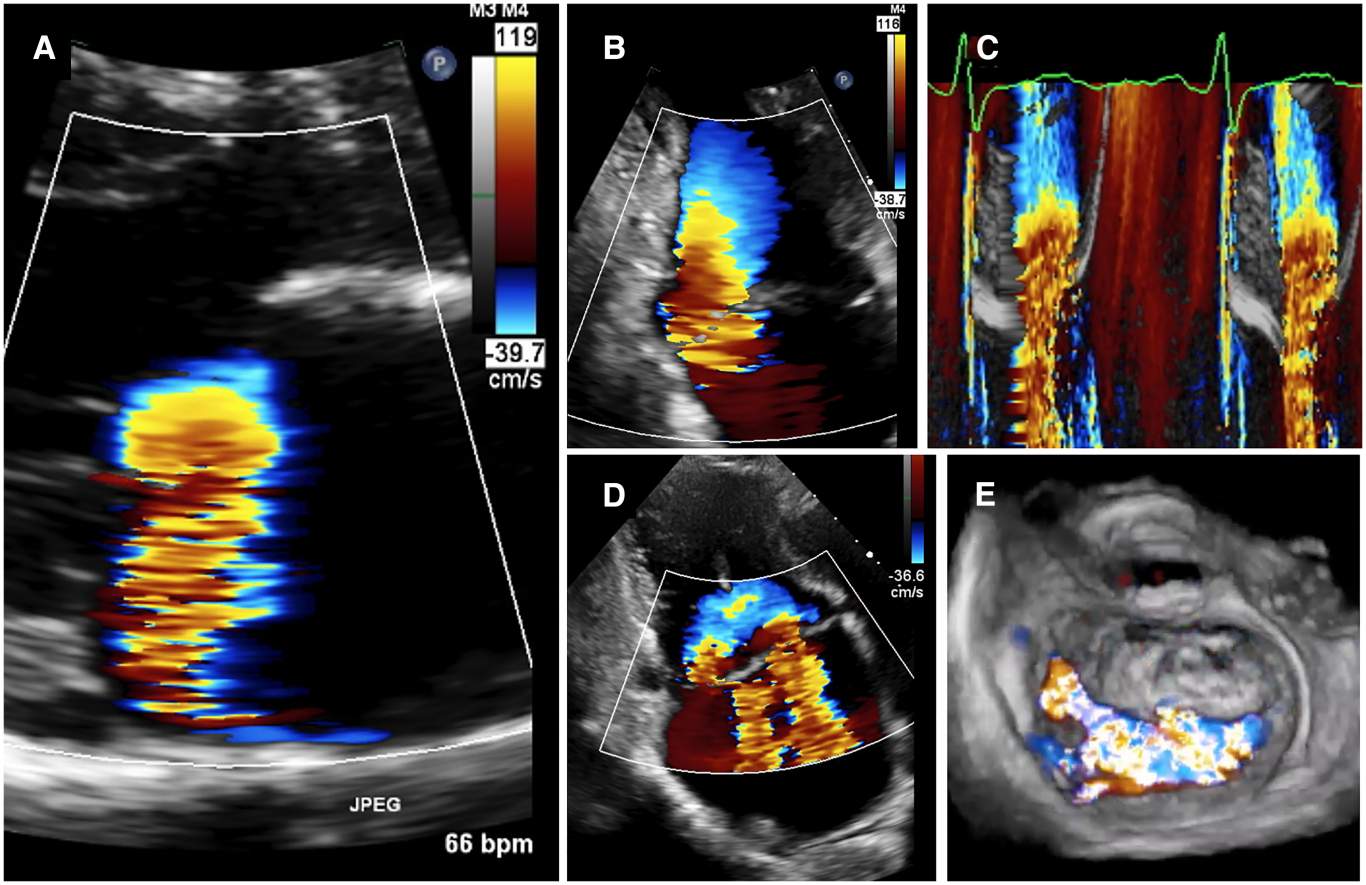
Examples of pitfalls of the PISA method. (A) Patient with anterior MV prolapse. A clear hemispheric-shaped proximal flow convergence is seen on parasternal long-axis view (A). However, this is not the most common situation encountered in clinical practice. (B) Wall-constrained proximal flow convergence with a oblong shape. This is frequently observed in case of posterior MV prolapse or commissural MR. There is a risk of over-estimation of MR EROA and RegVol by the PISA method. (C) Late-systolic MR. As shown in Doppler color coupled to M-mode, the MR is only present in the latter part of systole. Therefore the MR EROA which depends on a single time point measurement (the PISA radius) is not reliable to grade MR. (D) Multiple MR jets. The PISA method is not reliable to grade MR. Indirect quantitative methods should be considered. (E) Bi-leaflet prolapse with extensive MR from one commissure to another. The PISA method is inapplicable. Indirect quantitative methods should be considered. EROA, effective regurgitant orifice area; LV, left ventricular; MR, mitral regurgitation; MV, mitral valve; PISA, proximal isovelocity surface area.
